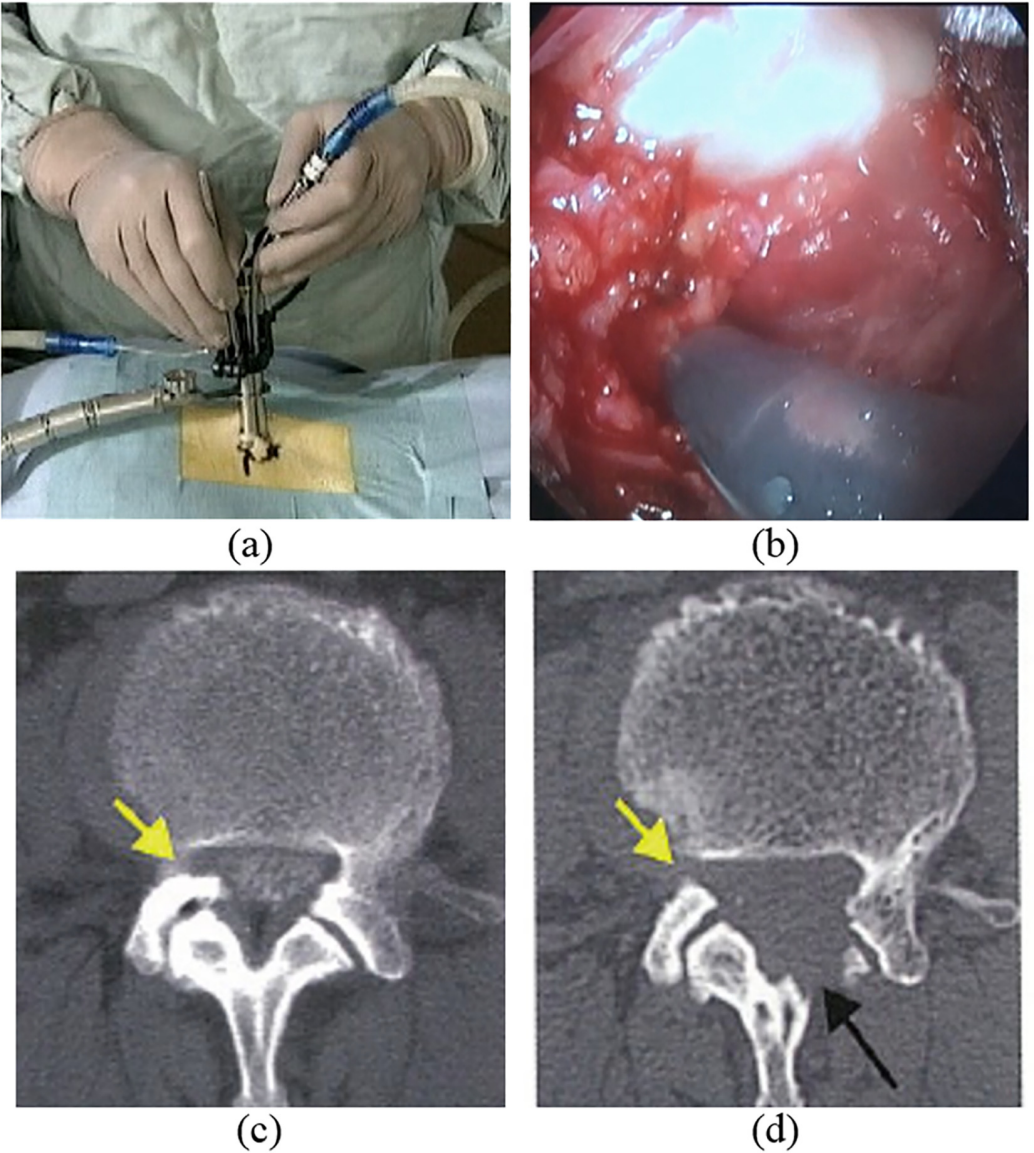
Microendoscopic discectomy for spinal disorders. (a) Tool operation during surgery. (b) Endoscopic view with a 16-mm diameter. (c) Preoperative and (d) postoperative CT images. The yellow and black arrows show the cutting results.
